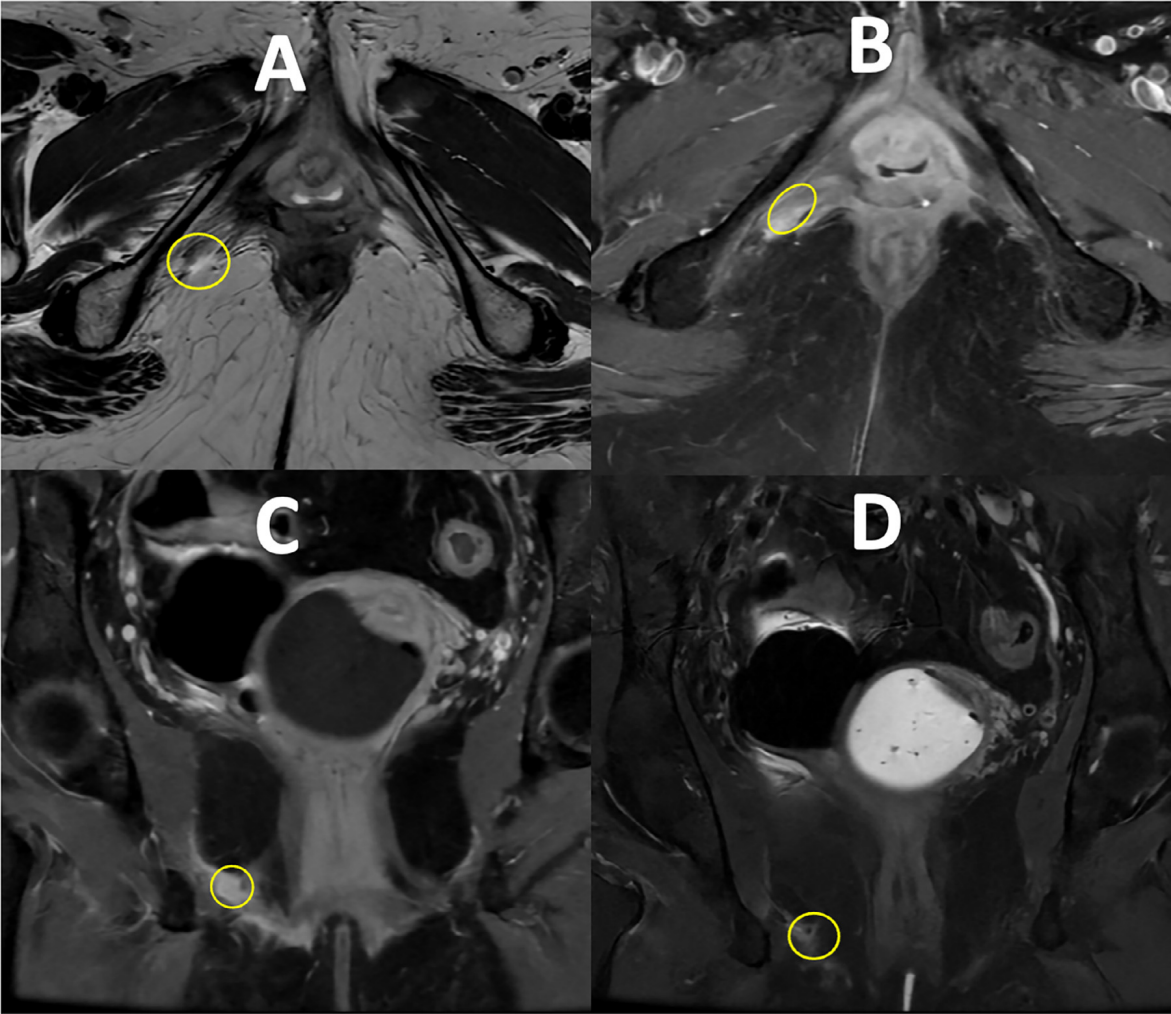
October 2024: MRI scan performed 15 months after SABR showed ongoing response of the lesion remaining isointense and no further contrast enhancement identified (A, T2w axial; B, T1w axial with contrast; C, T1w coronal with contrast; D, T2w fat-suppressed coronal).
